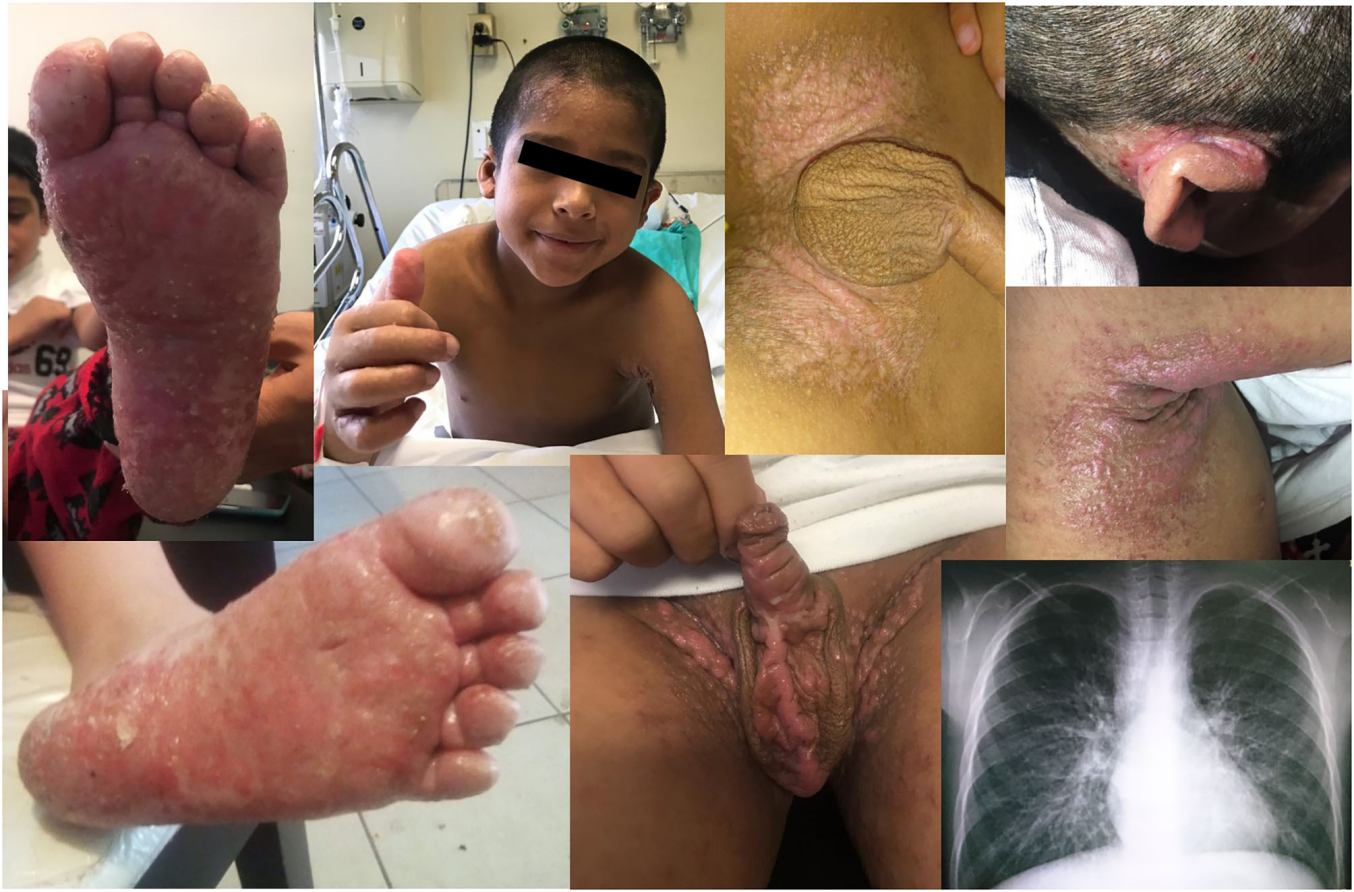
Some clinical features of DOCK8 deficiency. Plain warts and discolored skin patches in forehead, trunk, hands, and soles. Severe retroauricular and axillar eczema. Lower right corner: chest radiograph showing bilateral bronchiectases and perihilar interstitial infiltrates.
